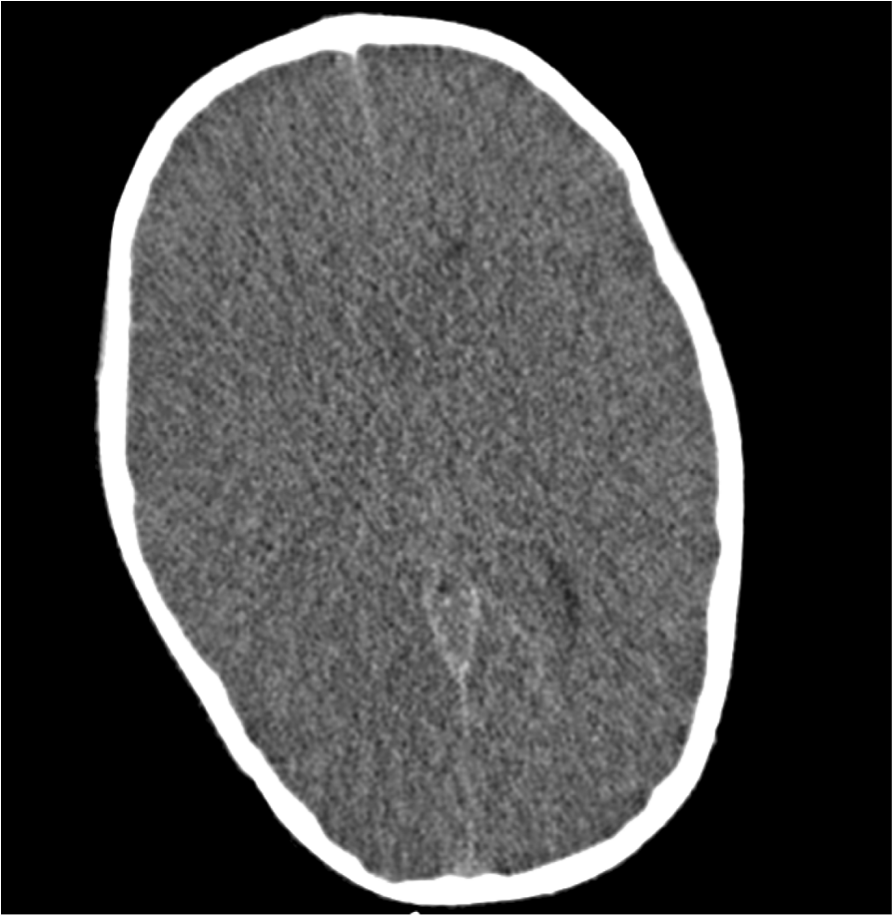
CT scan of the brain. Loss of cortical convolutions; reduction of cisternal cerebral spaces; reduced gray to white matter tissue intensity contrast.
